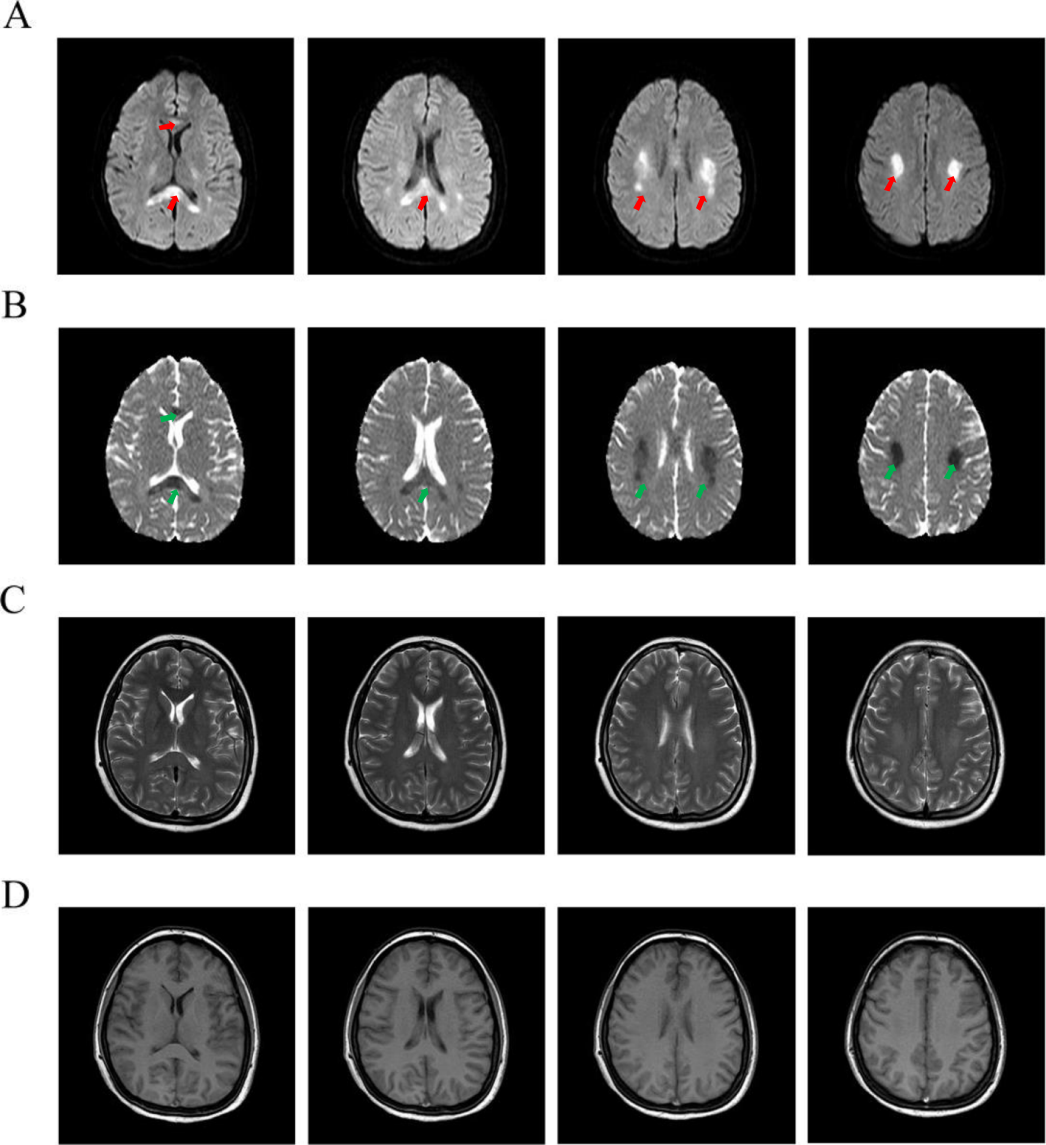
Axial MRI images of brain. (a) DWI images showed high signal intensity involving corpus callosum and radiata (red arrows). (b) ADC images indicated that the signal intensity was opposite to the DWI images in the same lesions (green arrows). T2-weighted axial images (c) and T1-weighted images (d) were normal
